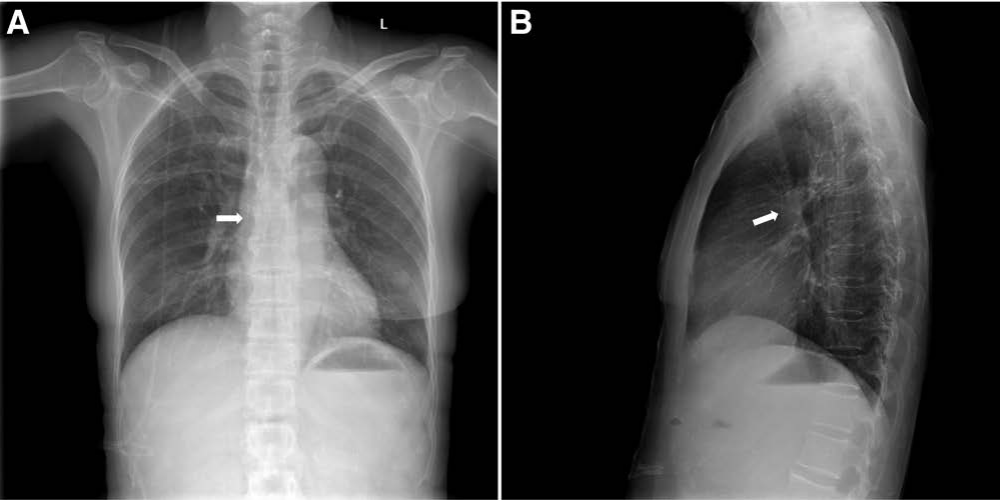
After the intervention, posterior/anterior and lateral chest X-rays of patient A confirmed that the tip of the PICC was restored to the lower third of the SVC (white arrows point to the tip of the PICC). PICC = peripherally inserted central catheter, SVC = superior vena cava.
